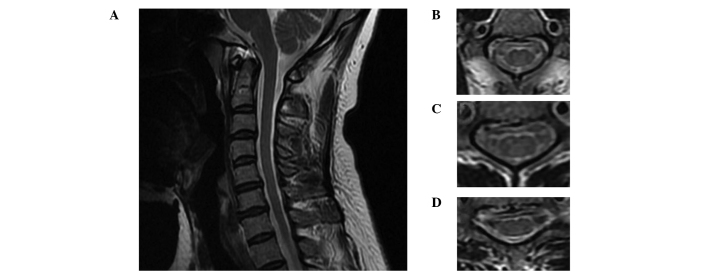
MRI scans of the spinal cord. (A) Sagittal T2-weighted MRI scan showing the normal appearance of the posterior column, despite a number of disc osteophyte complexes with mild central canal stenosis. Axial T2-weighted MRI scans at the (B) C2, (C) C4 and (D) C6 levels. MRI, magnetic resonance imaging.
